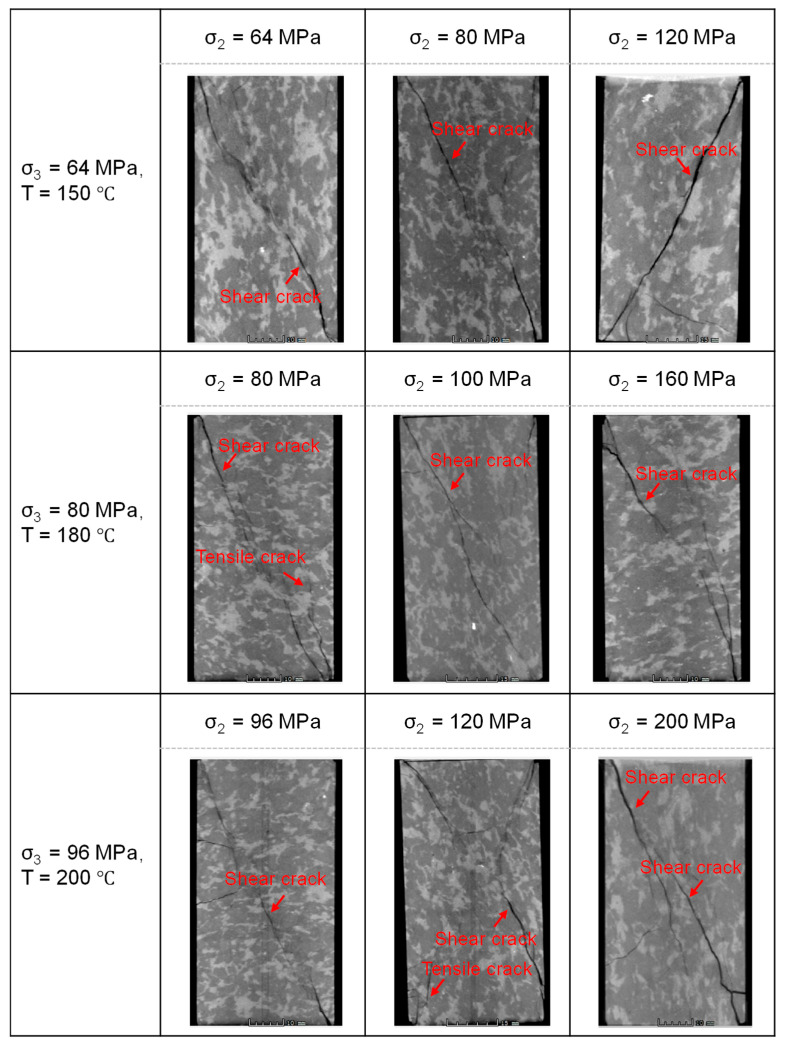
CT slices of granite after failure under different σ_2_ conditions (The sample in the CT slice is 40 cm wide and 80 cm high).
